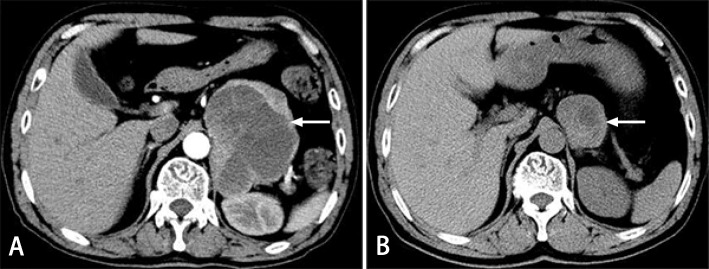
Abdominal computed tomography (CT) scan (a) shows a 9.6 × 8.3 cm left adrenal mass with an inhomogeneous density; lung CT scan (b) shows a 4.4 × 4.0 cm mass in the left adrenal area
